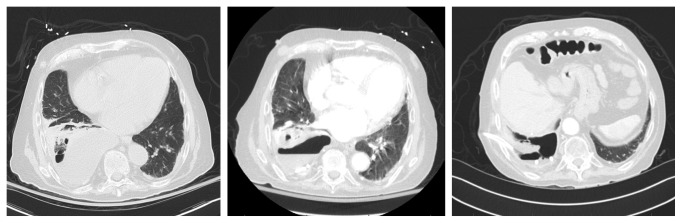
CT chest from admission, after chest tube placement, and at 1-month follow-up.
